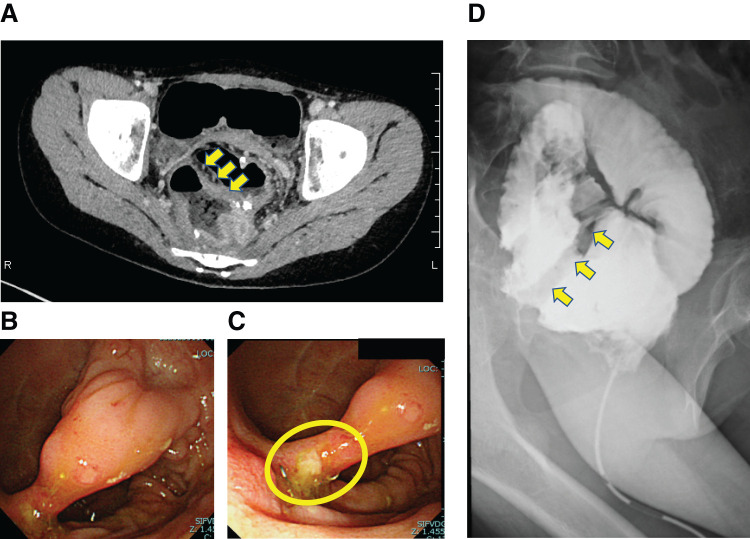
Preoperative image examination findings. (A) CT on admission showing what was interpreted as thickening of the wall of the ileal J-pouch. Retrospectively, this was recognized as a mucosal bridge with a stapler line at one end (yellow arrows). (B) Full endoscopic view of a mucosal bridge in the J-pouch. (C) Root of mucosal bridge. A stapler line can be identified at the root of the mucosal bridge (yellow circle). (D) Contrast enema image on admission. Interruption of contrast is apparent in the J-pouch (yellow arrows).
